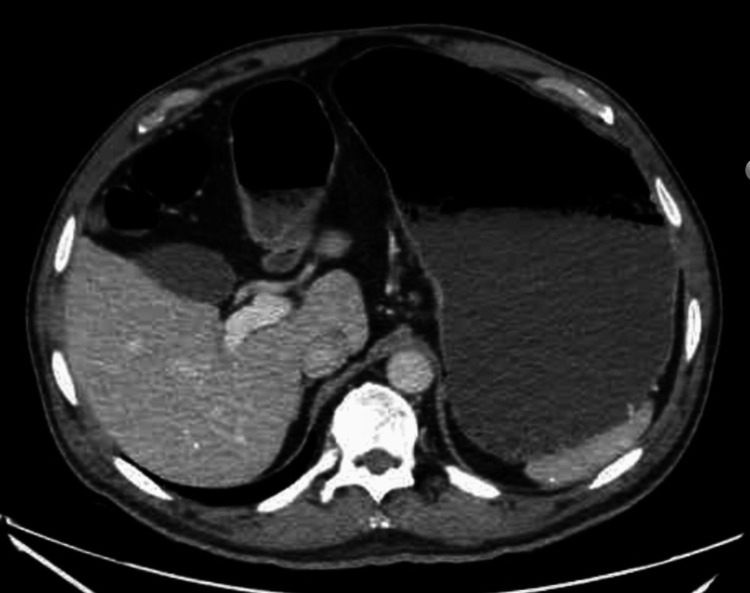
CT of the abdomen with contrast showing significant gastric distention and fluid retention
